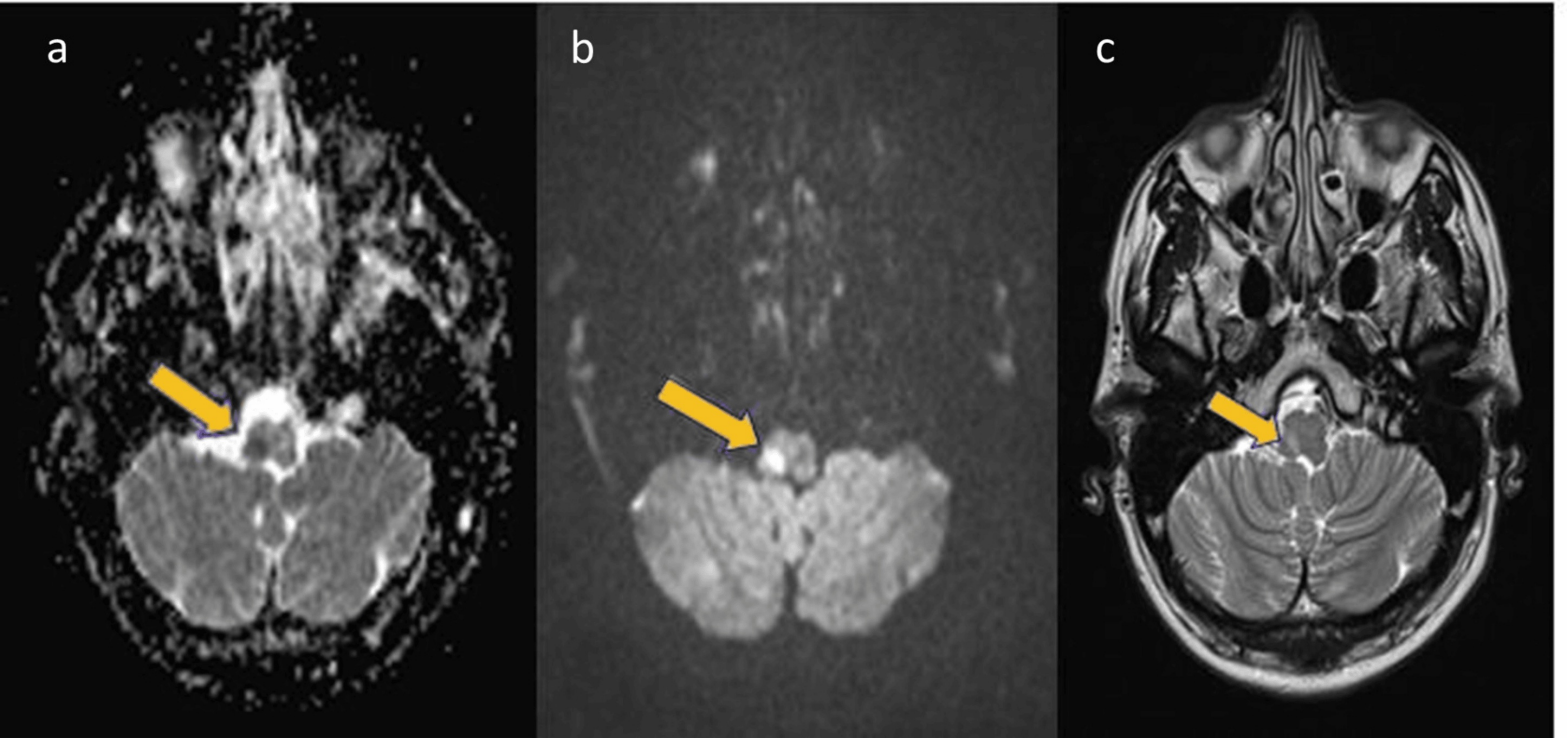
Brain magnetic resonance imaging showed an infra-centimetric acute ischemic lesion in the right posterolateral medulla. Diffusion-weighted (a), fluid-attenuated inversion recovery (b), and T2-weighted (c) imaging sequences.
